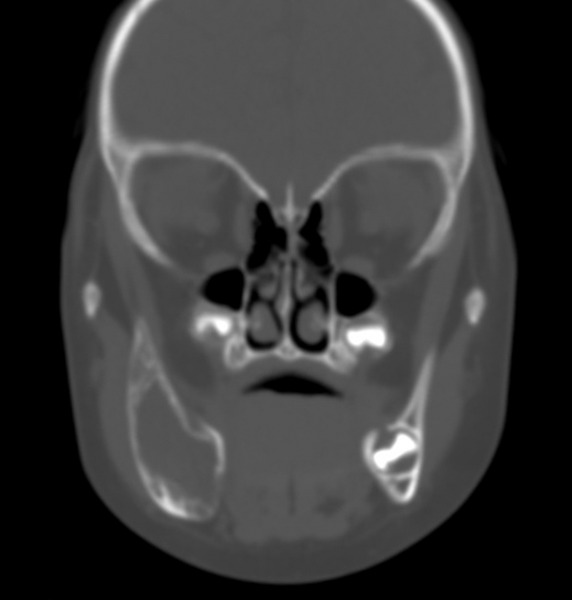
Computed tomography scan showing a suspicious expansive lytic lesion of the mandible with cortical erosion
